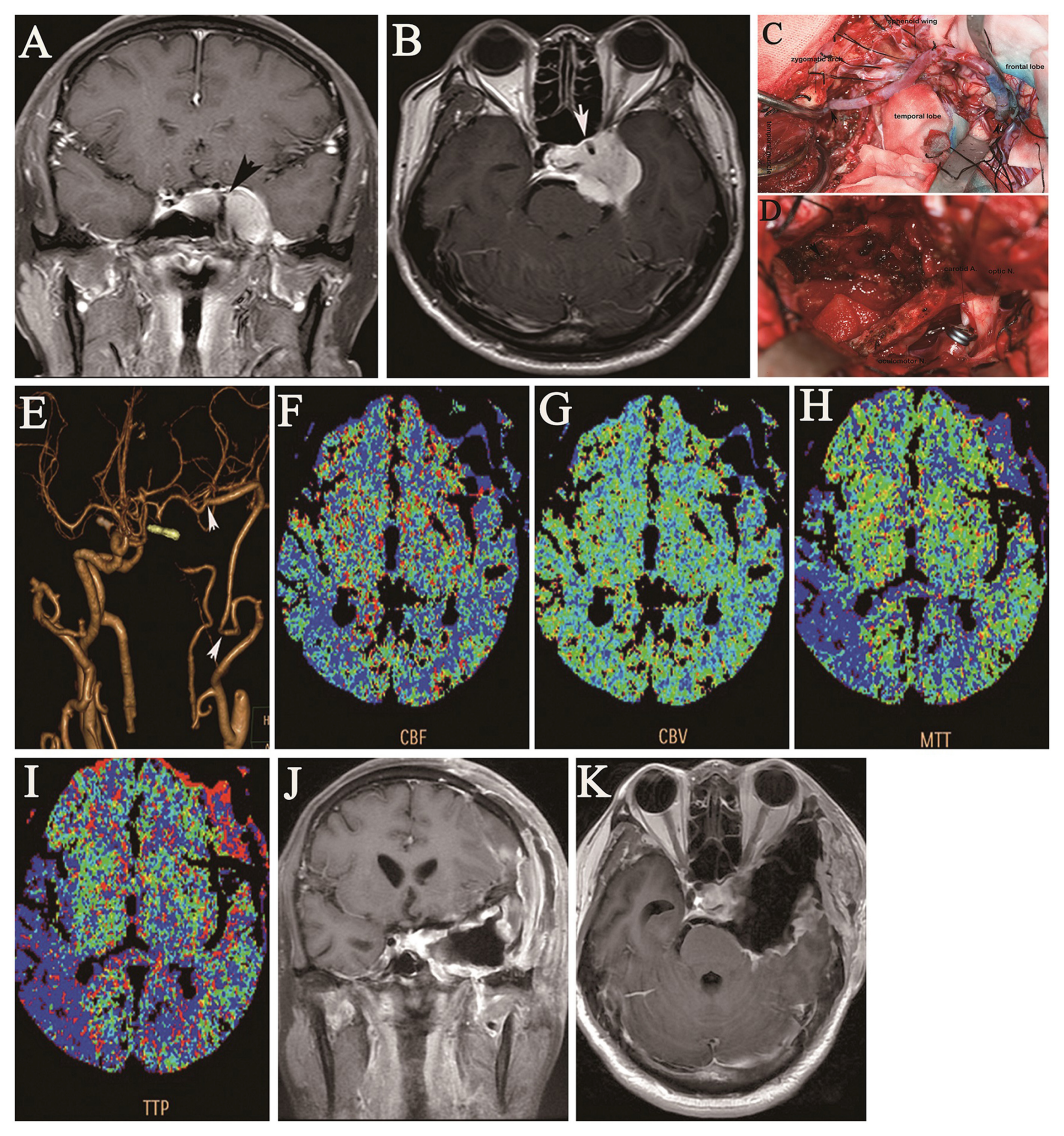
A 46-year-old man presented with a 7-year history of double vision and left-sided facial numbness for 1 year and ptosis for half a year. Coronal (A) and axial (B) preoperative contrast-enhanced MRI shows a large tumor in the cavernous region encasing the ICA (A), as indicated by the arrow. Intraoperative images show end-to-end anastomosis of the IMA to the RA and end-to-side anastomosis of the M2 segment of the MCA through a frontotemporal craniotomy with resection of the zygomatic arch (C), the operative field of the cavernous region (as indicated by the arrow), and the clipped supraclinoid ICA after total tumor resection (D). Postoperative CTA shows the patency of the IMA bypass with the RA graft and occlusion of the left ICA; the arrows indicate the anastomotic sites of the RA graft (E). Postoperative CT perfusion imaging shows the (F) cerebral blood flow, (G) cerebral blood volume, (H) mean transit time, and (I) time-to-peak for the left cerebral hemisphere after IMA bypass, which is consistent with symmetry in the hemodynamic capacity of the right cerebral hemisphere. Coronal (J) and axial (K) contrast-enhanced MRI at the 2-year follow-up shows no tumor growth in the cavernous region.
